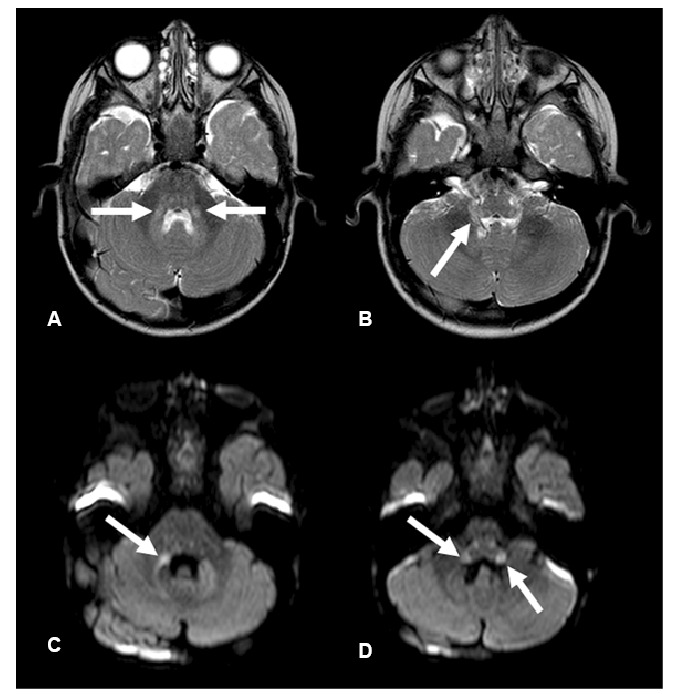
Brain magnetic resonance imaging demonstrates hyperintense lesions on T2-weighted imaging (A and B), with restricted diffusion (C and D), involving the pons and middle cerebellar peduncles (arrows in A and C), as well as the medulla and inferior cerebellar peduncles (arrows in B and D), consistent with rhombencephalitis.
